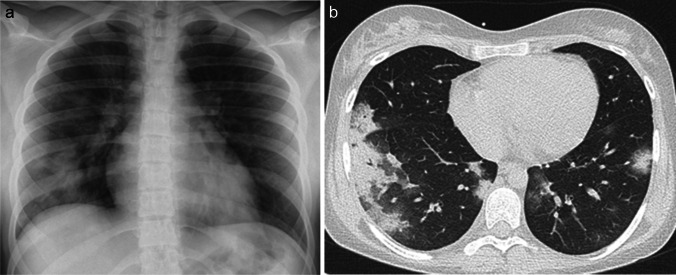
Images in a 17-year-old girl with coronavirus disease 2019 (COVID-19) who presented with fever, cough, and fatigue. a Anteroposterior supine chest radiograph shows bilateral peripheral opacities predominately in the basal segments of the lungs. b Axial non-contrast-enhanced lung window computed tomography image shows the typical signs of COVID-19 in children: subpleural and peripheral consolidations in basal and posterior lung segments surrounded by ground-glass opacities (the halo sign)
